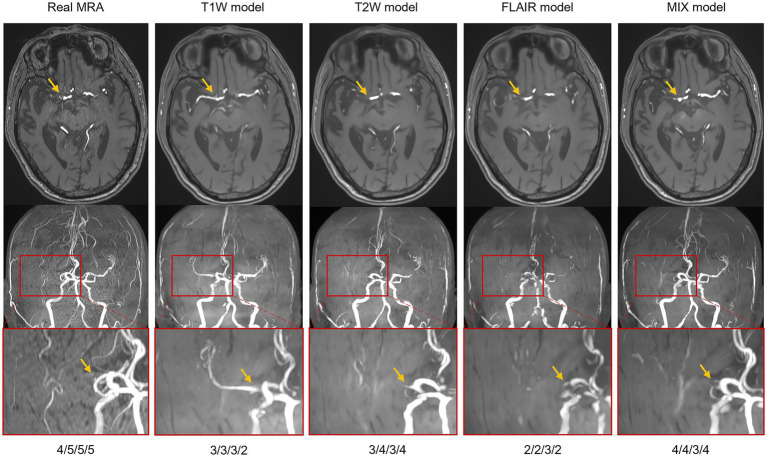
Comparison of synthetic MRA images generated by different Models. Male, 72 years old, real MRA showed occlusion of the right middle cerebral artery. T2W, FLAIR, and MIX model syn-MRA all accurately identified the abnormality, while T1W model syn-MRA showed a false negative at this location (yellow arrow). The red box indicates the magnified image, n/n/n/n means overall image quality/vascular edge sharpness/venous contamination/diagnostic confidence scores. MRA = magnetic resonance angiography, T1W = T1-weighted; T2W = T2-weighted, FLAIR = fluid attenuated inversion recovery.
